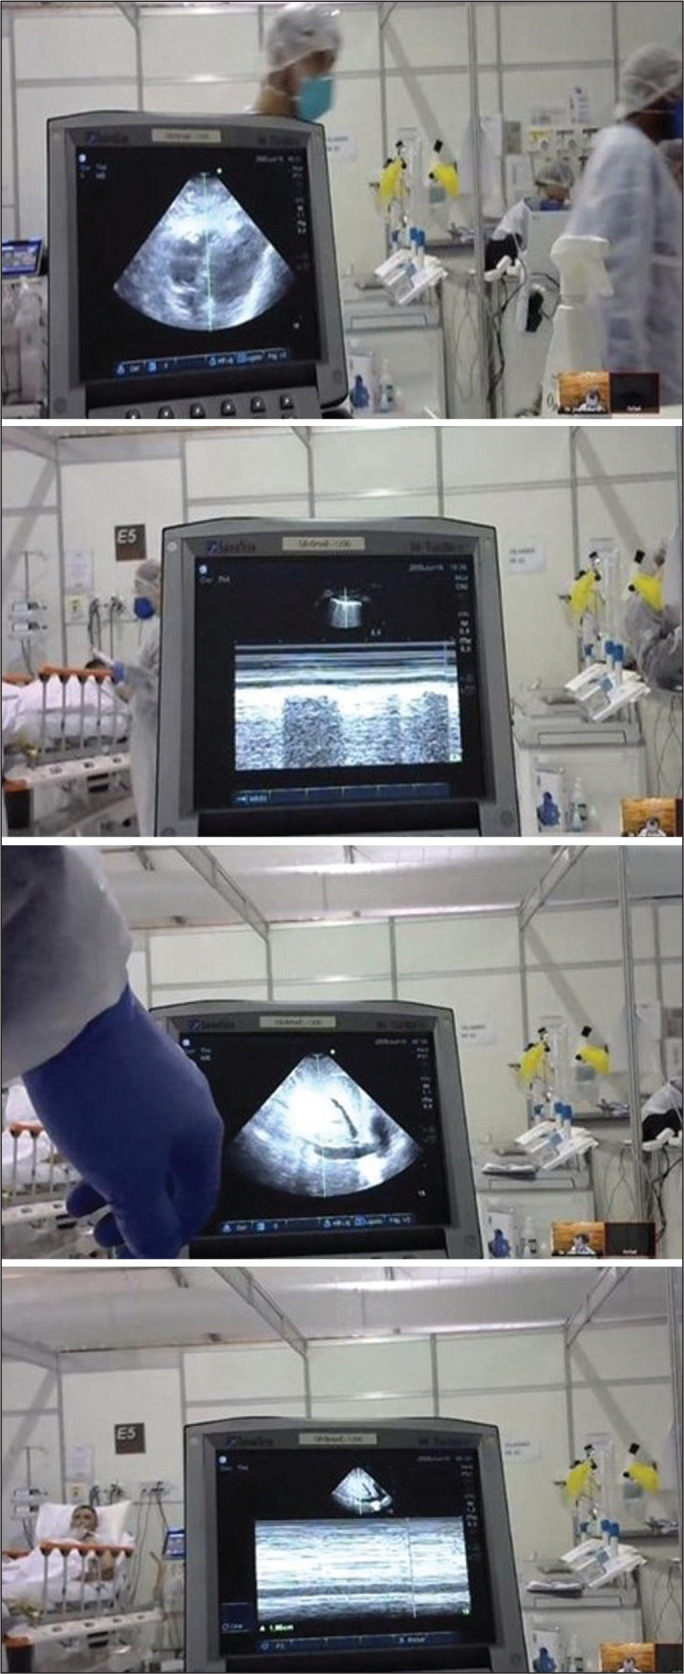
Screenshots of the telemedicine-guided bedside ultrasound performed at the field hospital.
